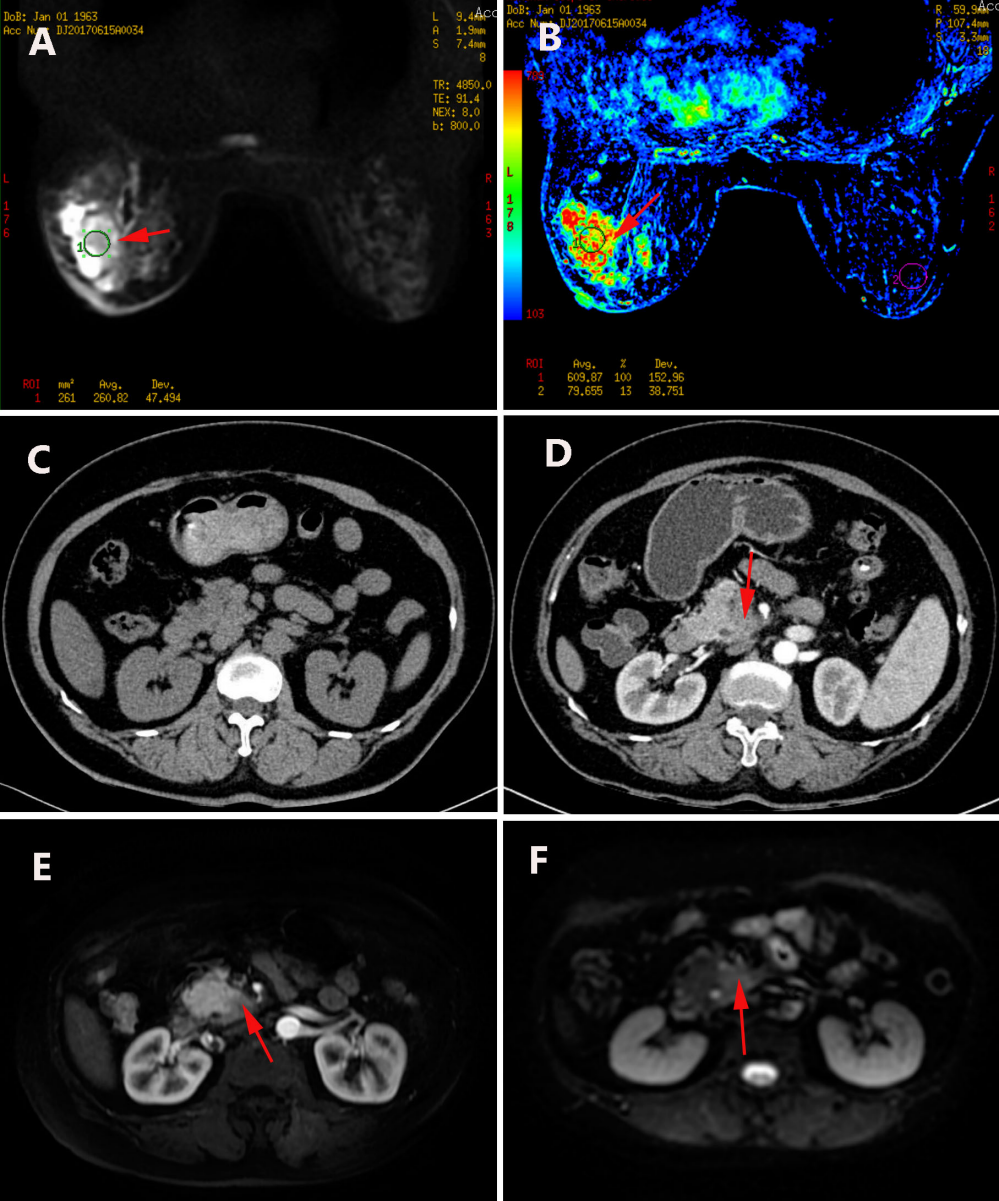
(A) Breast MRI demonstrates restricted diffusion in the left breast. (B) Parametric color map of the breast MRI. (C) Upper abdominal CT performed in 2017 shows no pancreatic abnormalities. (D) Upper abdominal CT in 2025 reveals a mass in the uncinate process of the pancreas. (E) Arterial phase of pancreatic MRI shows a hypodense lesion in the uncinate process. (F) Pancreatic MRI indicates restricted diffusion within the uncinate process. (The red arrow indicates the location of the tumor).
